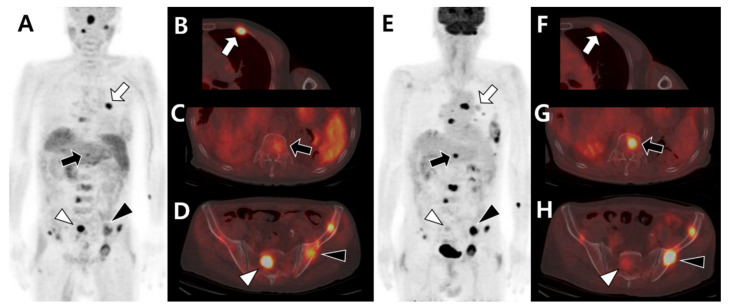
Different tracer avidities according to involved sites of bone metastases in the same patient with hepatocellular carcinoma before therapy. ^11^C-acetate positron emission tomography (PET)/computed tomography (CT) (A–D) shows intense acetate uptake in metastatic lesions of the left third rib (white arrow in A,B) and sacrum (white arrowhead in A,D) but mild acetate uptake in the T12 vertebra (black arrow in A,C) and left iliac bone (black arrowhead in A,D). ^18^F-fluorodeoxyglucose (^18^F-FDG) PET/CT (E–H) shows mild FDG uptake in the left third rib (white arrow in E,F) and sacrum (white arrowhead in E,H) but intense FDG uptake in the T12 vertebra (black arrow in E,G) and left iliac bone (black arrowhead in E,H).
